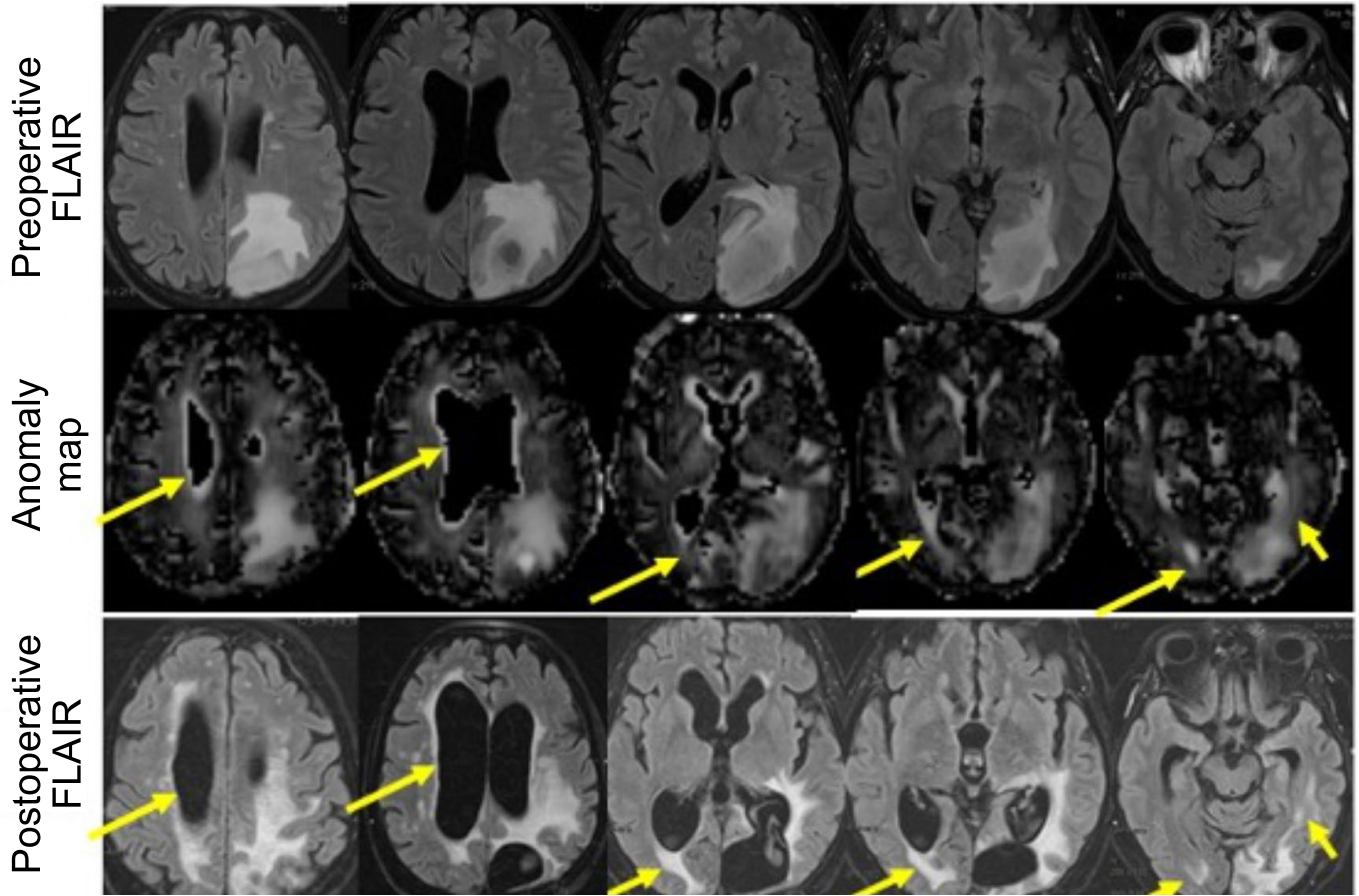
Areas with high anomaly score show up on follow-up scans. Upper two rows: FLAIR-weighted MRI and diffusion anomaly detection-based data obtained preoperatively. Lower row: FLAIR-weighted MRI 18 months later. Yellow arrows mark areas in which tumor recurrence was observed and in which the anomaly score was higher than expected based on the preoperative FLAIR scan.
